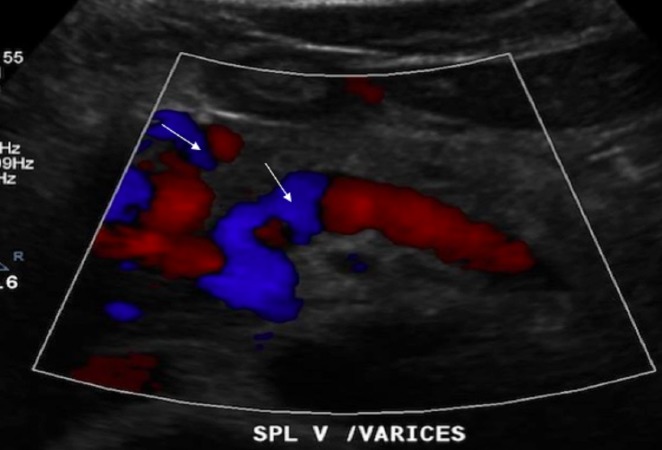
Doppler ultrasound of the liver revealing multiple venous collaterals (white arrows) seen in the porta hepatis, consistent with cavernous transformation
